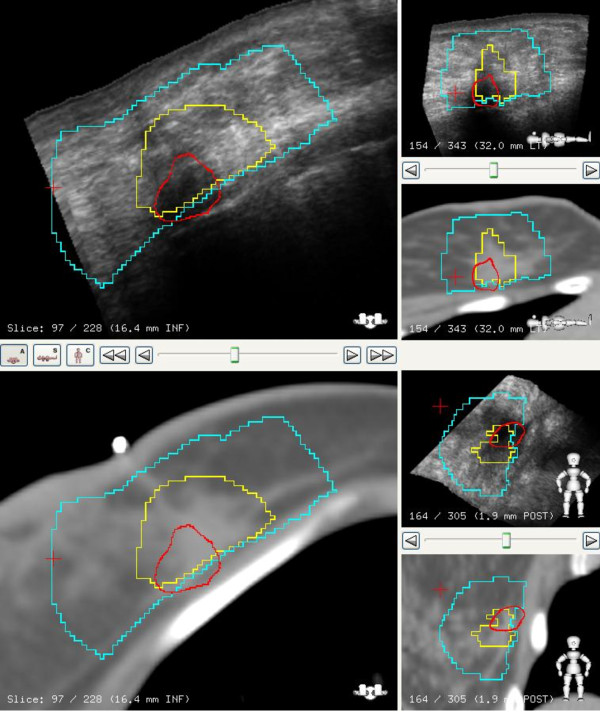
US and CT image co-registration in axial, sagittal and coronal views. The CT cavity (GTV) is in blue, US seroma (RV) in green, and the blue represents PTV. The RV is not always in the geometric center of the GTV.
